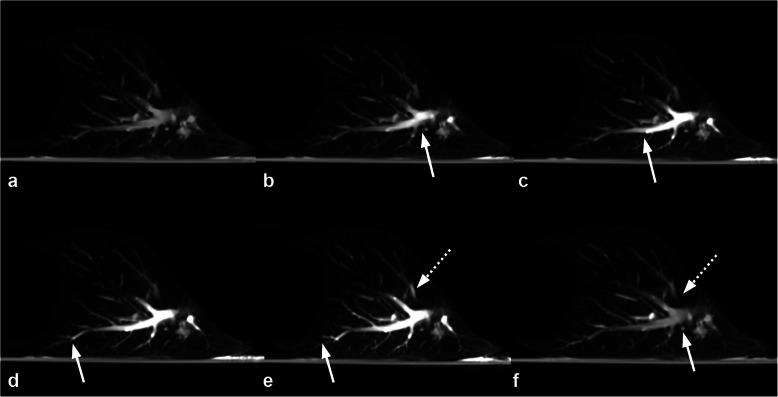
Computed tomography perfusion imaging of case #3 at t30. While unenhanced pulmonary vessels can be observed prior to contrast media injection (a), a gradual filling of the arterial vessels can be observed (white arrow, from b to e), followed by a filling of the pulmonary veins (dotted arrow in e), and a complete washout of the contrast agent
